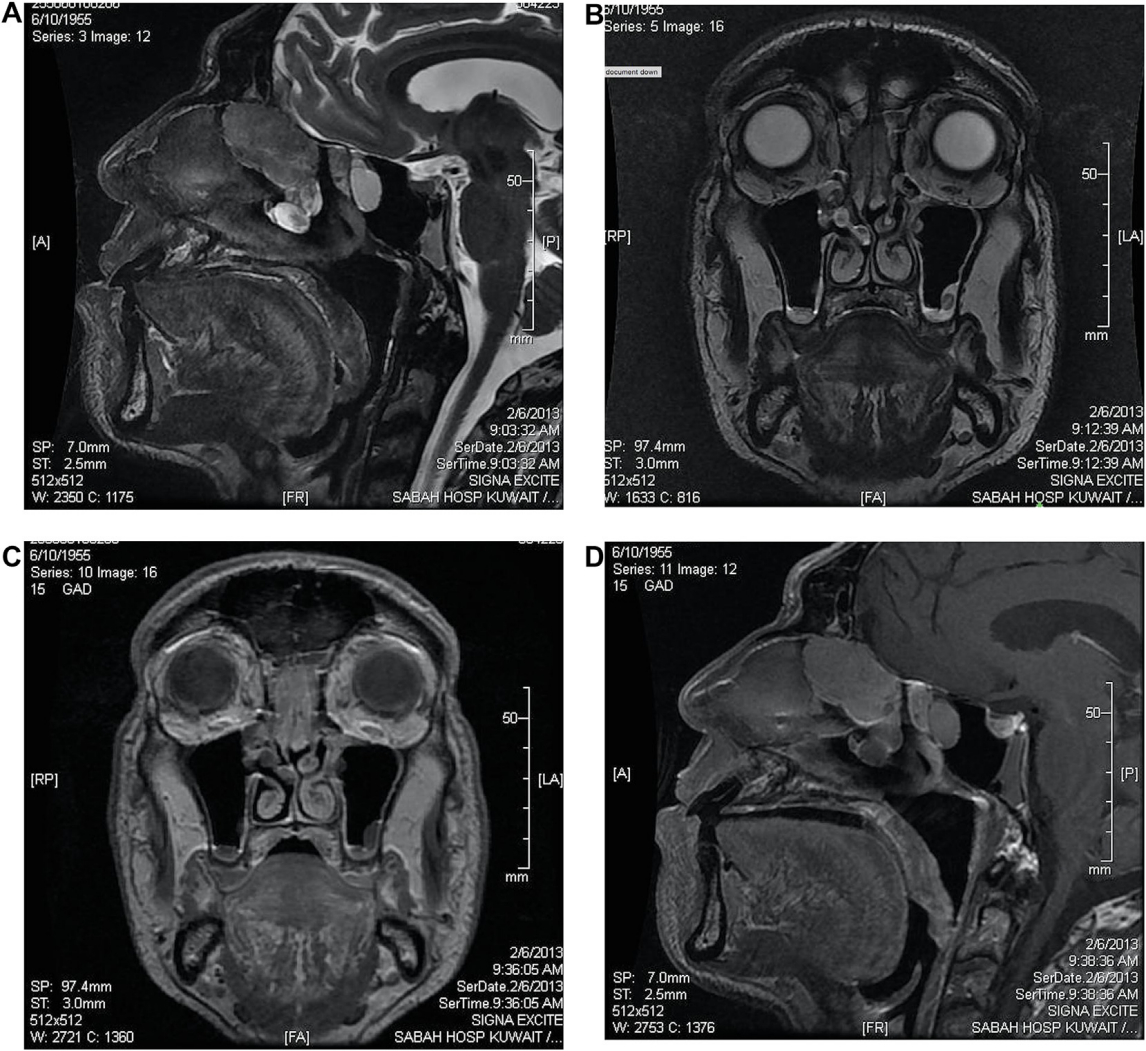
A, B, C, & D MRI sinus showing the olfactory recess bilaterally with soft tissue pathology extending to skull base with no intra-cranial extension, showing intermediate signal intensity on T1 post contrast enhancement (A: sagittal, B:coronal) and T2 (C: coronal, D: Sagittal). suggestive of nasal roof mass lesion query neuroblastoma for biopsy.
